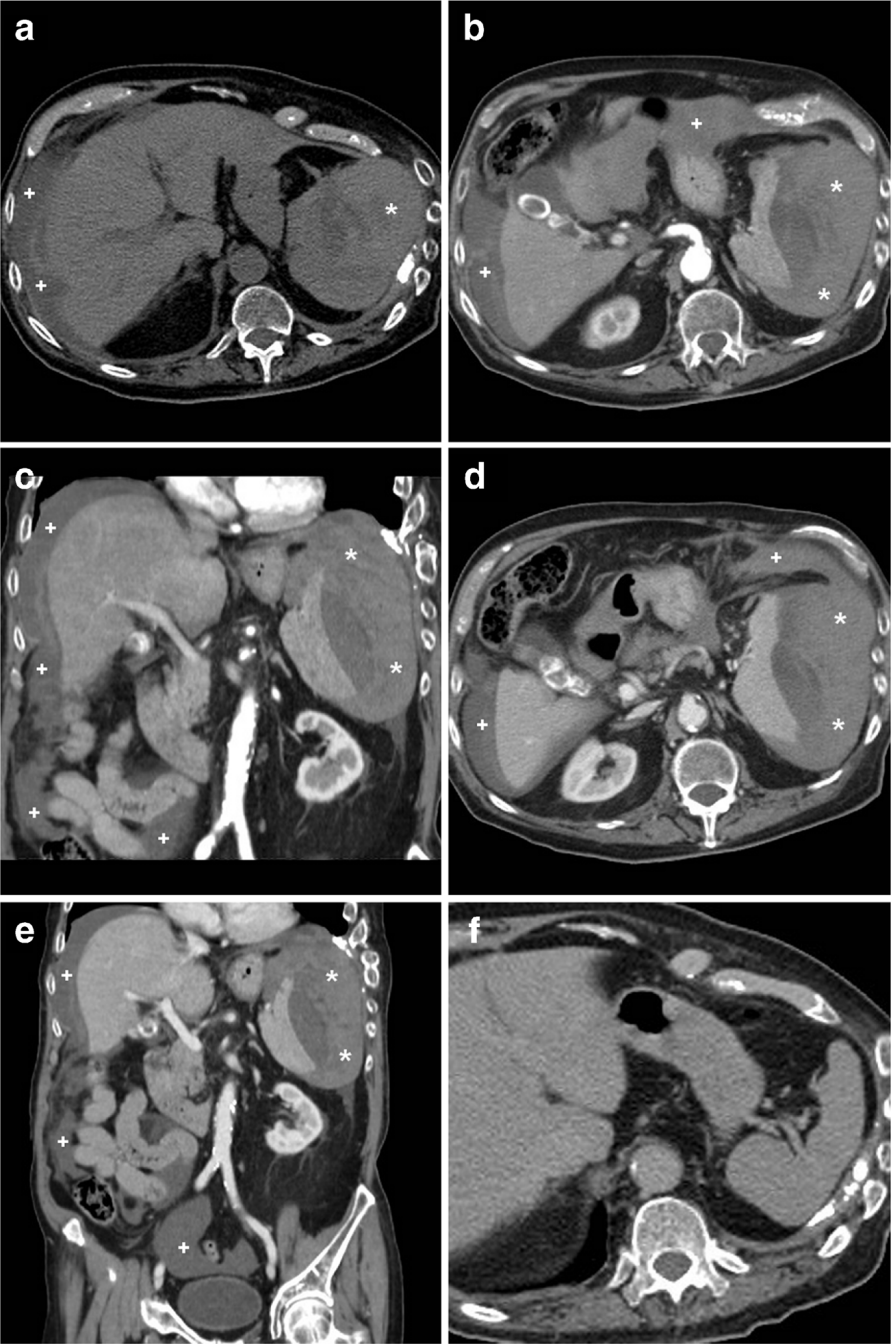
An elderly 89-year-old male with chronic heart failure, previous transurethral resection of non-muscle-invasive urinary bladder carcinoma, and lung emphysema experienced sudden hypotension and fainting. He was not on anticoagulants. Physical findings included palpation tenderness in the left hemiabdomen, tachycardia, and hypotension. Laboratory tests revealed severe blood loss: haemoglobin dropped from 9.2 to 6.4 g/dl within four hours. Platelet count, prothrombin time, and activated partial thromboplastin time were within normal range. Urgent CT including unenhanced (a), arterial- (b, c) and venous-phase (d, e) post-contrast images revealed mixed attenuation peritoneal effusion (+) consistent with haemoperitoneum. The spleen was surrounded, medially dislocated, and compressed by massive, fresh hyperattenuating (up to 55 Hounsfield units), partly subcapsular haemorrhage (*). Pseudoaneurysms and active bleeding were not seen. The spleen showed homogeneous parenchymal enhancement without focal lesions or signs of diffuse infiltrating disease. Retrospectively, contrast-enhanced CT obtained four months earlier (F) for bladder cancer staging revealed a normal, homogeneous spleen. The patient underwent urgent splenectomy and eventually recovered. Surgical pathology revealed medium-sized spleen with reactive lymphoid hyperplasia, and excluded acute infectious or neoplastic changes. Final diagnosis was idiopathic splenic rupture
